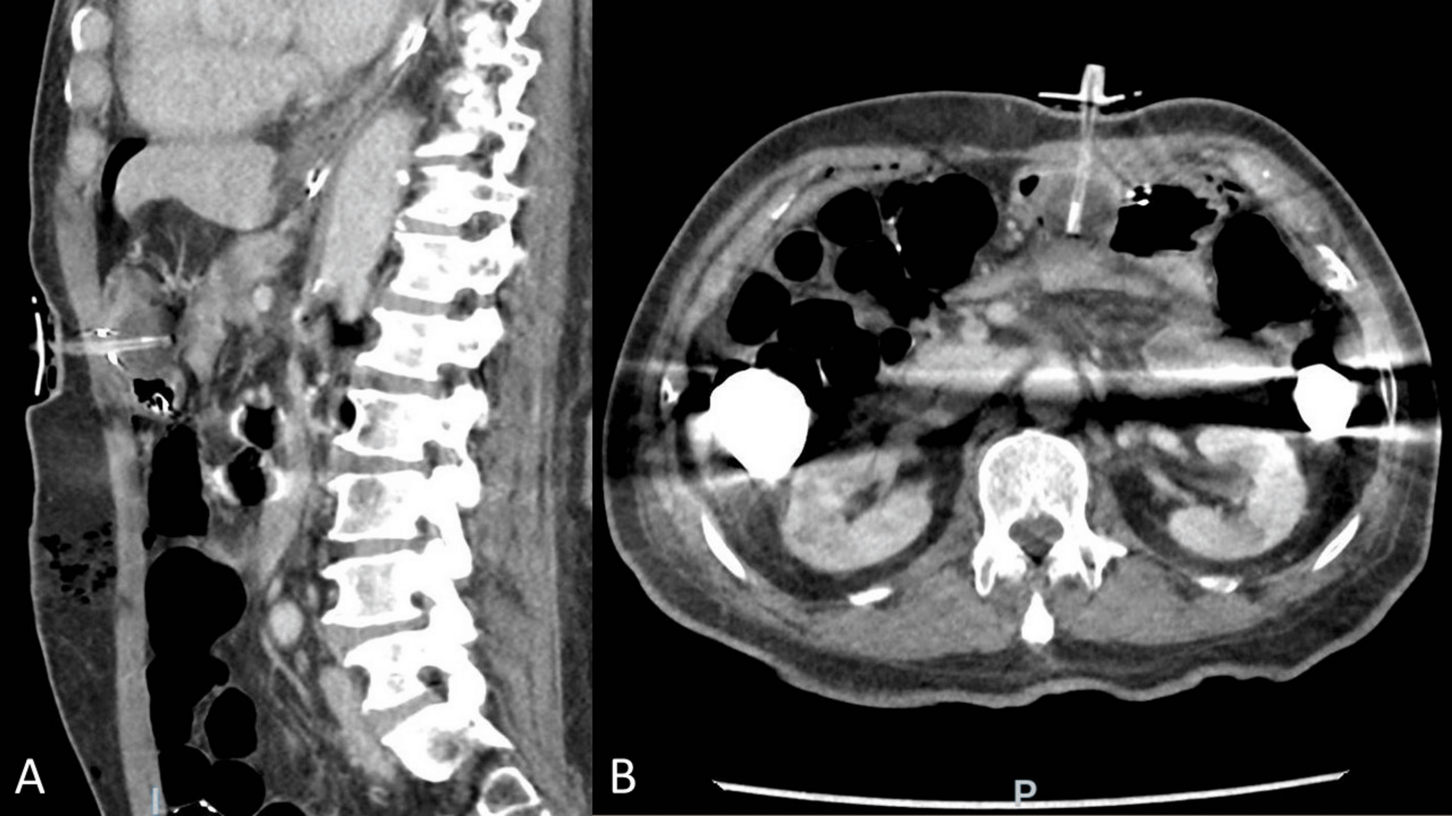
Computed tomography (A) sagittal and (B) transverse/axial images showing postoperative changes after laparoscopic gastrostomy tube placement through anterior and posterior walls of the stomach.
